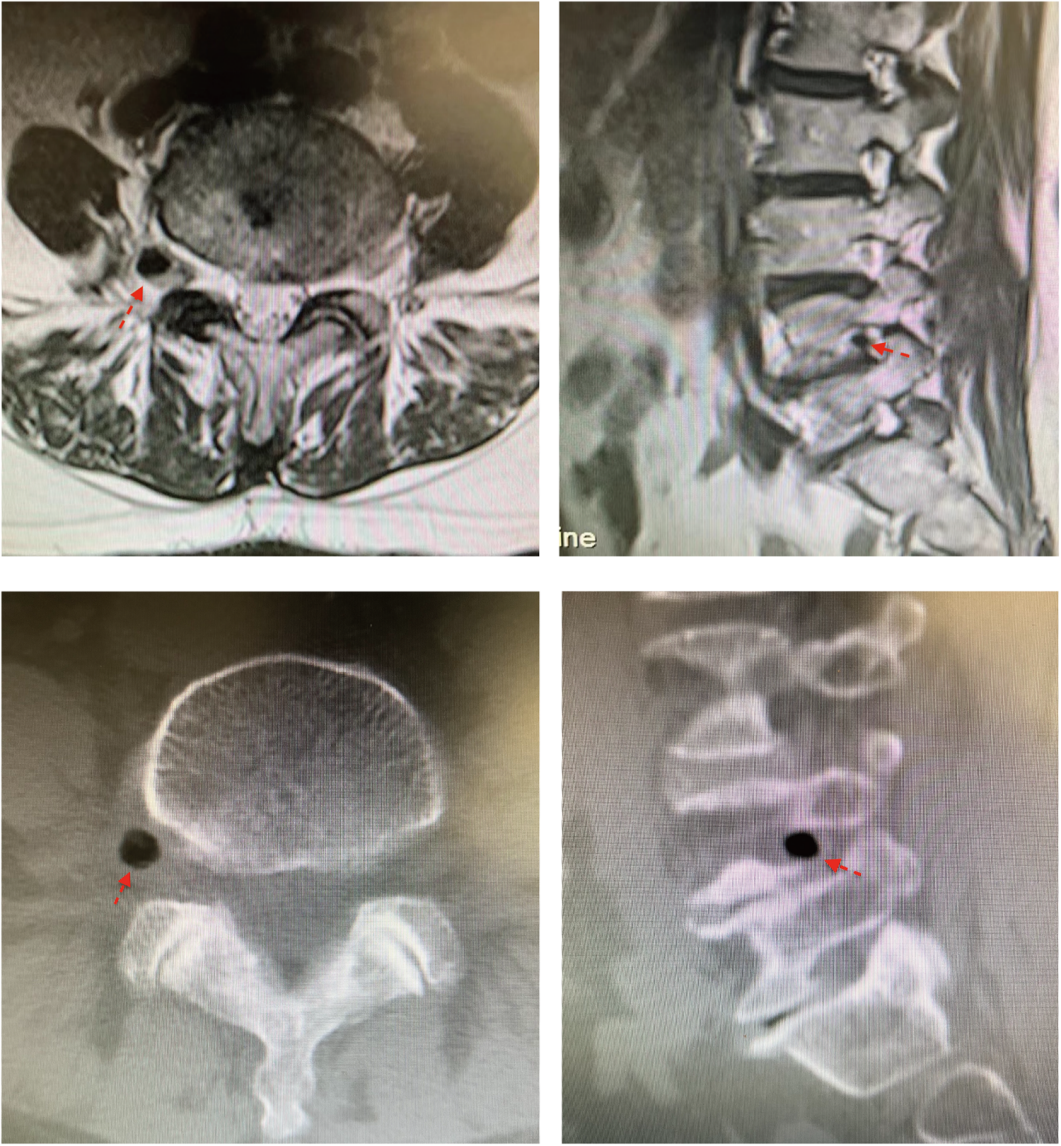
Right extraforaminal GCP of L4–5 was shown in MRI and CT. (A) MRI axial views of L4–5; (B) MRI sagittal view of L4–5; (C) CT axial views of L4–5; (D) CT sagittal view of L4–5. Red arrow indicated the extraforaminal GCP.
